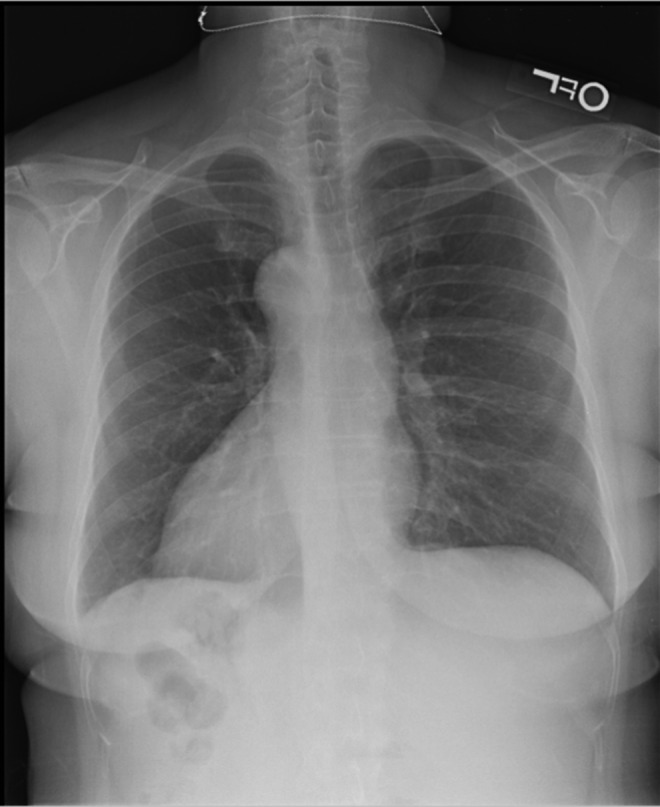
Chest radiograph shows dextrocardia.
